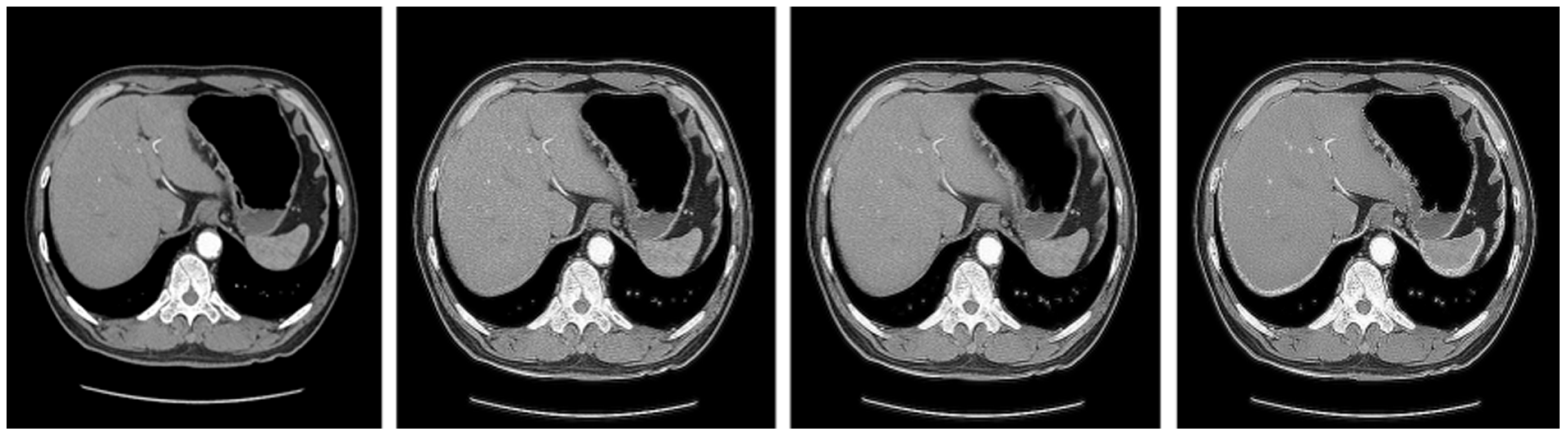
Shows comparison of restoration effect of three low-rank models in dealing with the CT images that contain large noise. From left to right: the 10^th^ original stomach CT image, the result of RPCA model, the result of LADMAP model, the result of GoDec model, we can see our method can do some good effect on the medical CT images which contain large noise, the contrast of the images after restoration enhance obviously and the human organ boundaries are clear, there is more detailed information shown out, but the recovery image based on GoDec model shows less noise than the other two images.
